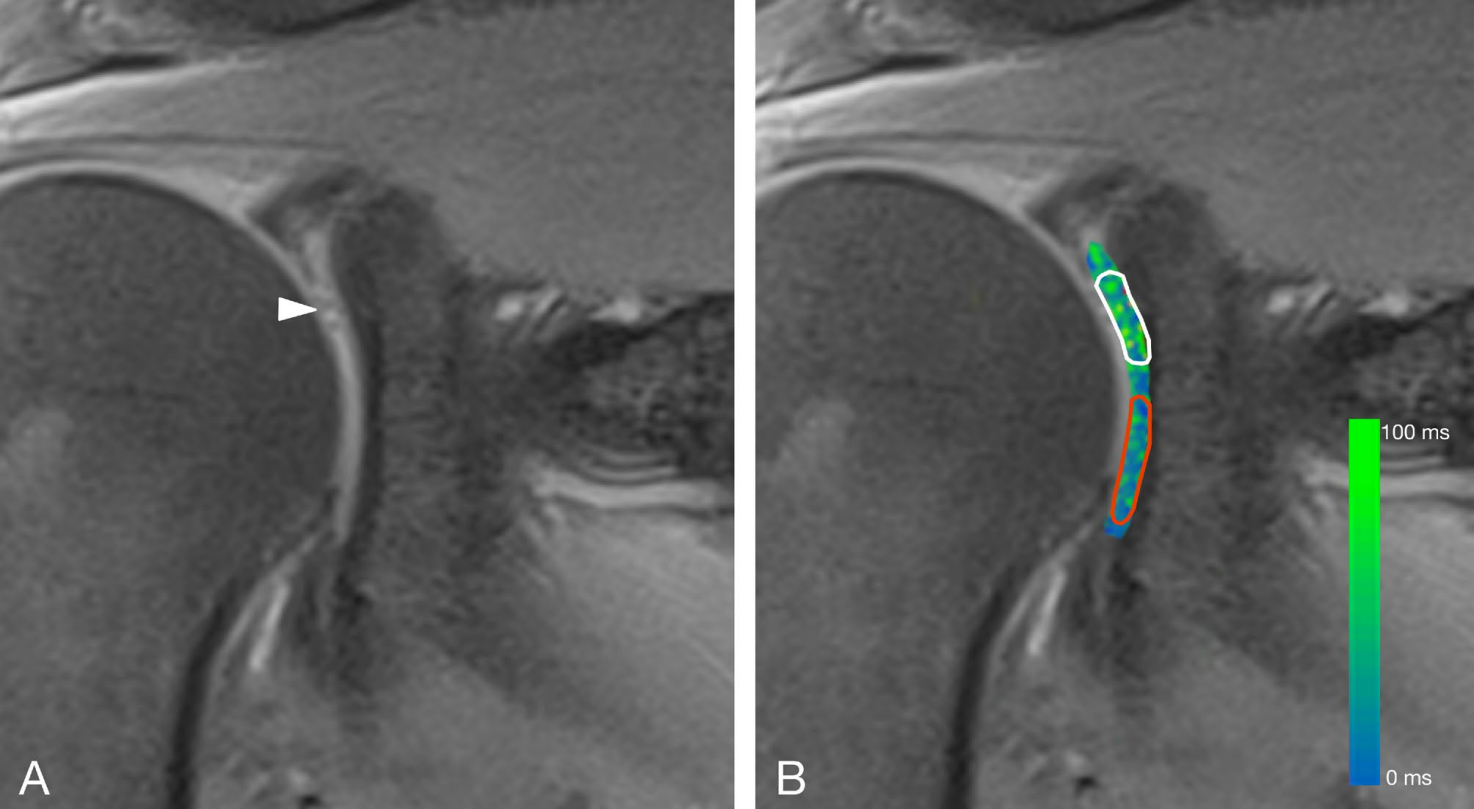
(A) Coronal proton-density fat saturated magnetic resonance image of a 55-year old female patient with a focal cartilage defect in the anterosuperior quadrant (white arrowhead). (B) Coronal proton-density fat saturated magnetic resonance image merged with the corresponding color-coded T2 map. The average T2 mapping value in the area of the focal cartilage defect was 40.8 ms (white ROI). The average T2 mapping value in the anteroinferior glenoid cartilage (red ROI) was 22.1 ms (Reader 1, Read 1). The average T2 mapping value of normal cartilage in the anterosuperior quadrant (ROI not shown) was 25.73 ms (Reader 1, Read 1).
